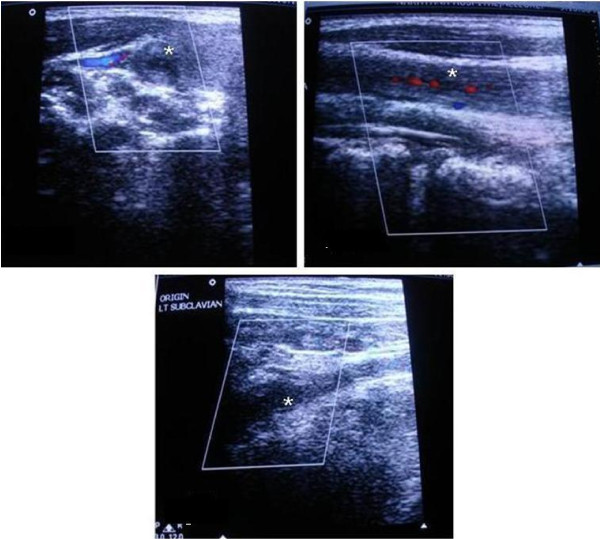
Color Doppler images of right (A) and left (B) common carotid arteries (*) show diffuse wall thickening and a streaky flow in the lumen. The origin of left subclavian artery (C) shows no flow.
